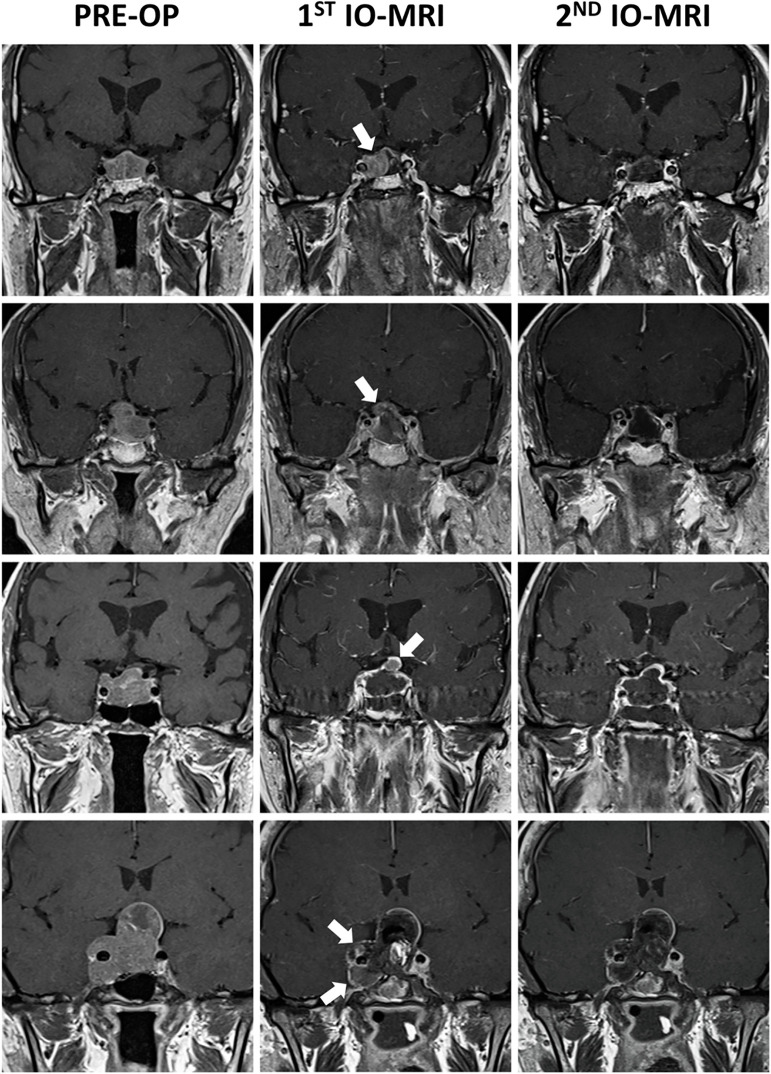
Figure demonstrating pre-operative and intraoperative contrast enhanced coronal T1-weighted pituitary MRIs of 4 different patients. First patient (first row) had a prolactin secreting macroadenoma which had two different signal intensity on pre-operative MRI scan without any cavernous invasion. Right side of the tumor was showing high signal intensity. First IO-MRI scan demonstrating residual mass on the right side. Second IO-MRI scan demonstrating that total removal was achieved. Second patient (second row) had a non-functioning macroadenoma causing visual disturbance. First IO-MRI scan demonstrating residual mass where tumor was penetrating the sellar diaphragm. Surgeon decided further excision and total removal was achieved. Third patient (third row) had a Knosp Grade 3A growth hormone secreting macroadenoma. Following excision, surgeon’s decision was in favor of total removal. However, first IO-MRI scan showed residual tumor was not in the cavernous sinus but in the region where tumor was penetrating the sellar diaphragm. Total removal was achieved following further excision. Last patient (fourth row) had a Knosp Grade 4 non-functioning pituitary adenoma causing severe visual disturbances. Following excision first IO-MRI demonstrated residual mass in the cavernous sinus. Further excision was performed which resulted in total removal of the tumor. Patient had a 3^rd^ nerve palsy following the surgery which totally improved after 3 months post-operatively. White arrows demonstrating residual tumor tissues on IO-MRIs.
